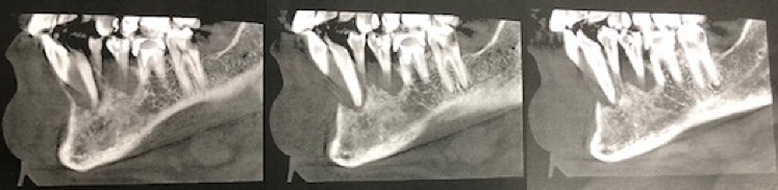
cone beam computed tomography, sagittal slices
